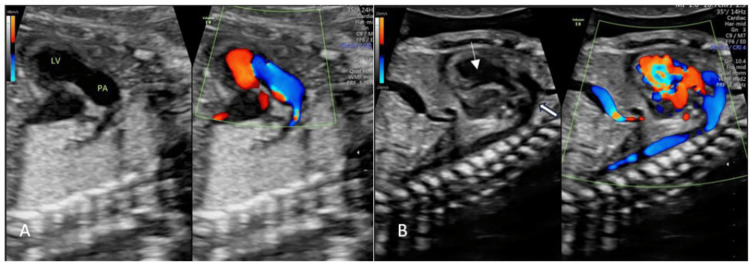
Color Doppler imaging A. The pulmonary artery originating from the morphological left ventricle (LV); B. The aorta (white arrow) originating from the morphological right ventricle (RV) (thin arrow)
